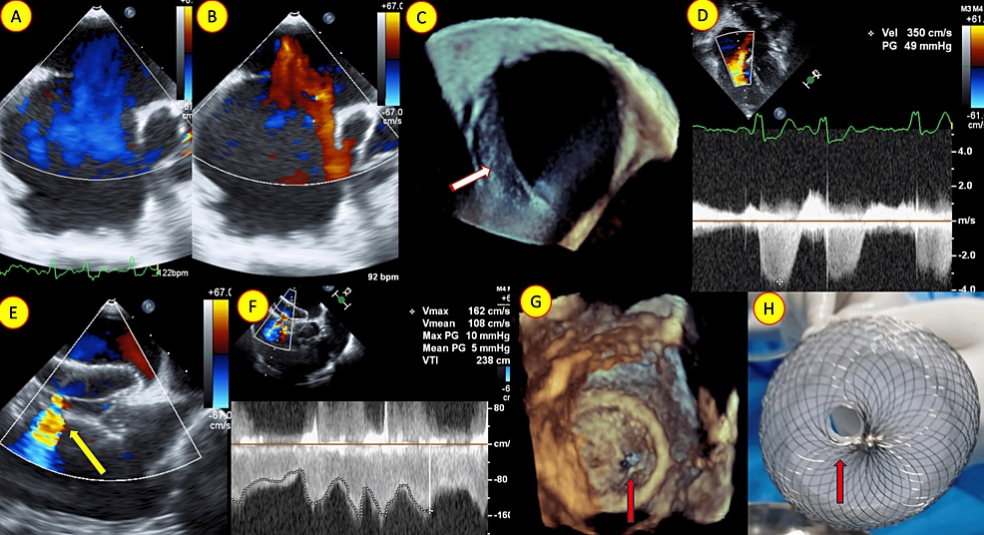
Transesophageal echocardiogram (TEE) in zero-degree view with color Doppler showing (A) left-to-right and (B) right-to-left shunt. (C) Three-dimensional enface TEE view showing the secundum atrial septal defect (ASD) with a thin floppy posterior inferior margin (white arrow). (D) The tricuspid regurgitation gradient is seen in the apical four-chamber view. (E) TEE in 90-degree view showing the atrial septal occluder in a stable position and the custom-made fenestration (yellow arrow) flowing left to right. (F) The continuous wave Doppler of the fenestration flow had a mean gradient of 5 mmHg. (G) Transesophageal three-dimensional enface view from the right atrial side showing the atrial septal occluder with the fenestration (red arrow). (H) The custom-made fenestration (red arrow) in the 40 mm Lifetech atrial septal occluder is shown.
